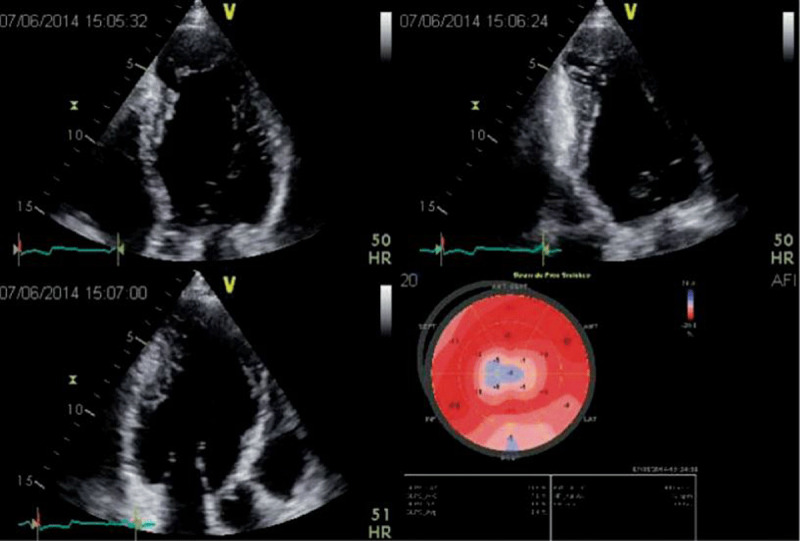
2D apical 4, 2 and 3-chamber views in a patient with Chagas disease and a typical aneurysm. In the 2-chamber view, a basal inferior aneurysm is also present. Longitudinal strain is abnormal in the apex, as well as on the basal inferior wall. Image: Marcia Barbosa. Reproduced with permission of the photographer.
